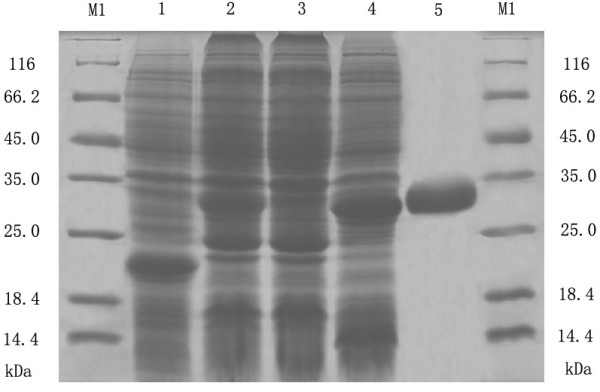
Expression and purification of recombinant protein. M1: Protein Marker; 1: Total protein from pET-32a (+) after induction; 2: Total protein from recombinant plasmid PET32a(+)-UL45Δ after induction; 3: Uninduced control; 4: The clear supernatant after ultrasonic disruption; 5: Purified recombinant UL45Δ protein using a single chromatographic step of IMAC on Ni ^2+ ^-NTA agarose.
